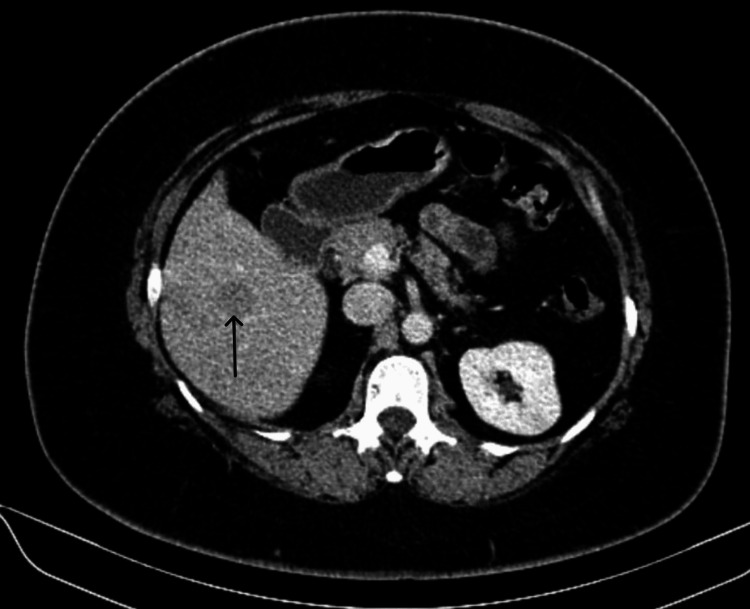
Contrast-enhanced computed tomography scan portal phase axial cut showing a lesion in the segment 6 (black arrow) of the liver.
